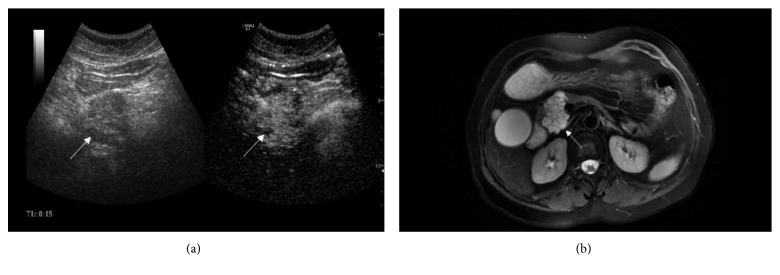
A 66-year-old woman with SCN in the pancreatic head. (a) CEUS shows the lesion is microcystic, the septa inside are enhanced, and microcysts are nonenhanced. (b) On T2-weighted MR image, the multiple septa show low signal.
